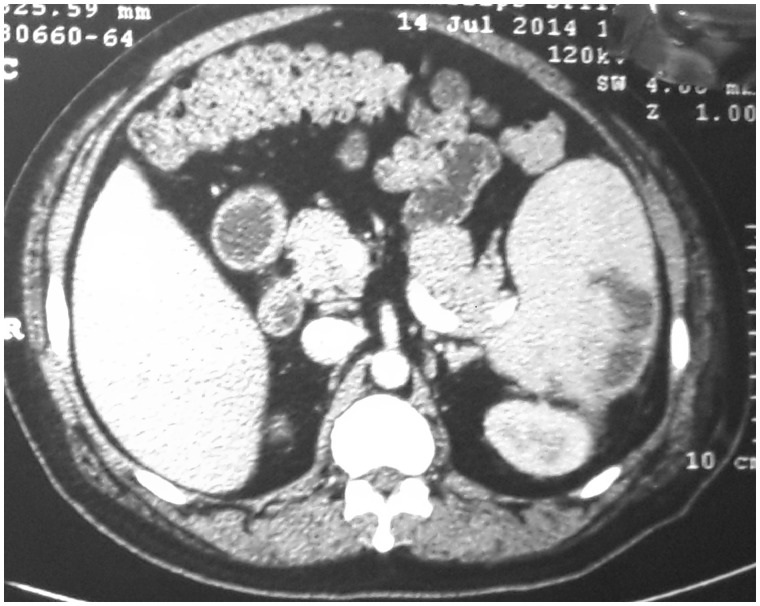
Contrast-enhanced spiral CT scan showing splenomegaly with multiple hypodense cystic lesions with ill-defined margins
